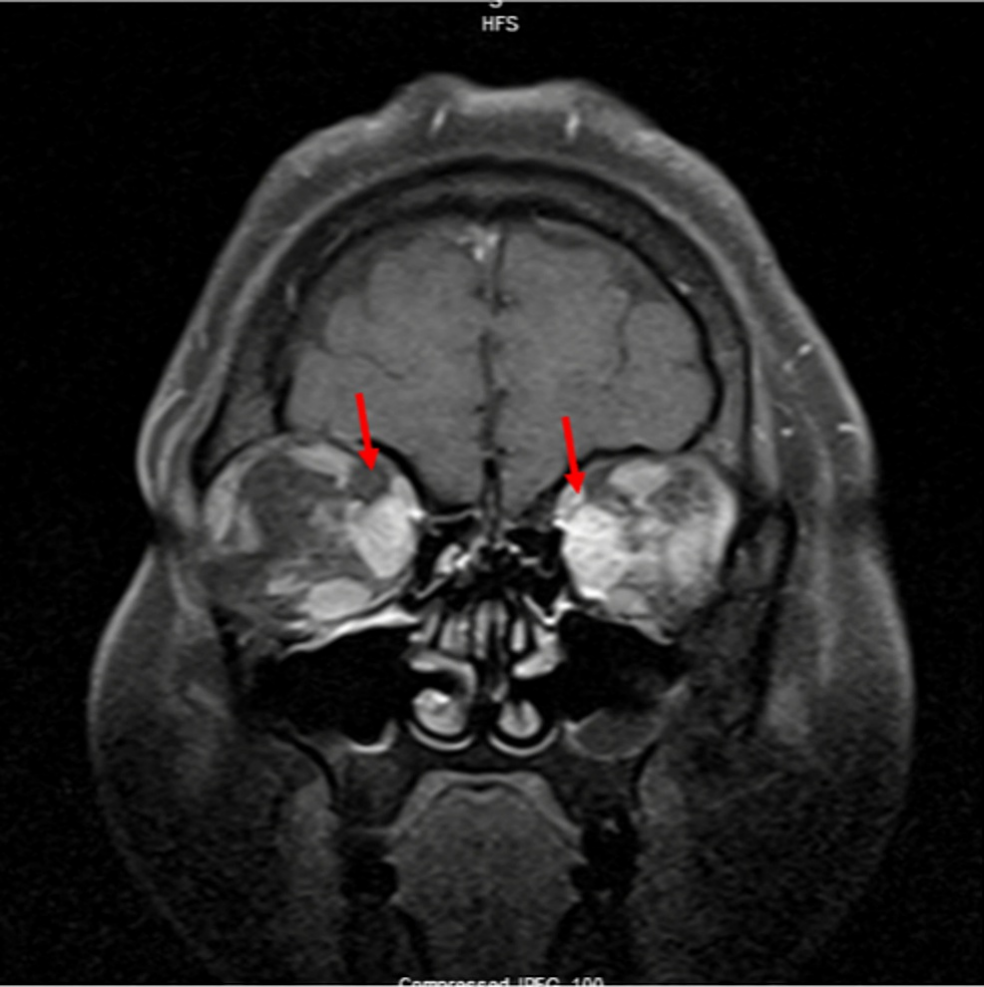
Orbital MRI (coronal view) shows another view of bilateral proptosis and ocular muscle hypertrophy indicated by red arrows. MRI, magnetic resonance imaging
